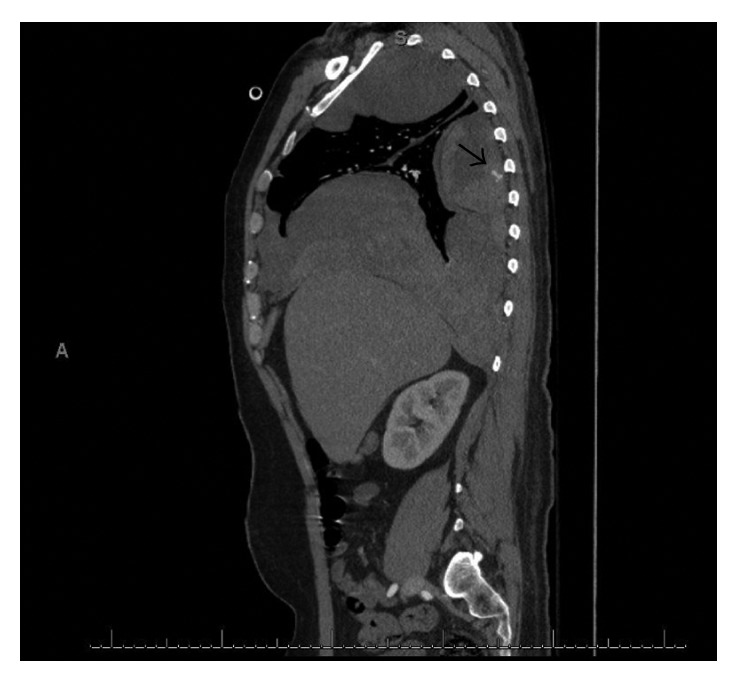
Arterial phase of CT chest scan in sagittal plane shows blushes of contrast (arrow) extending into the hematoma at the level of 7th posterior rib.
